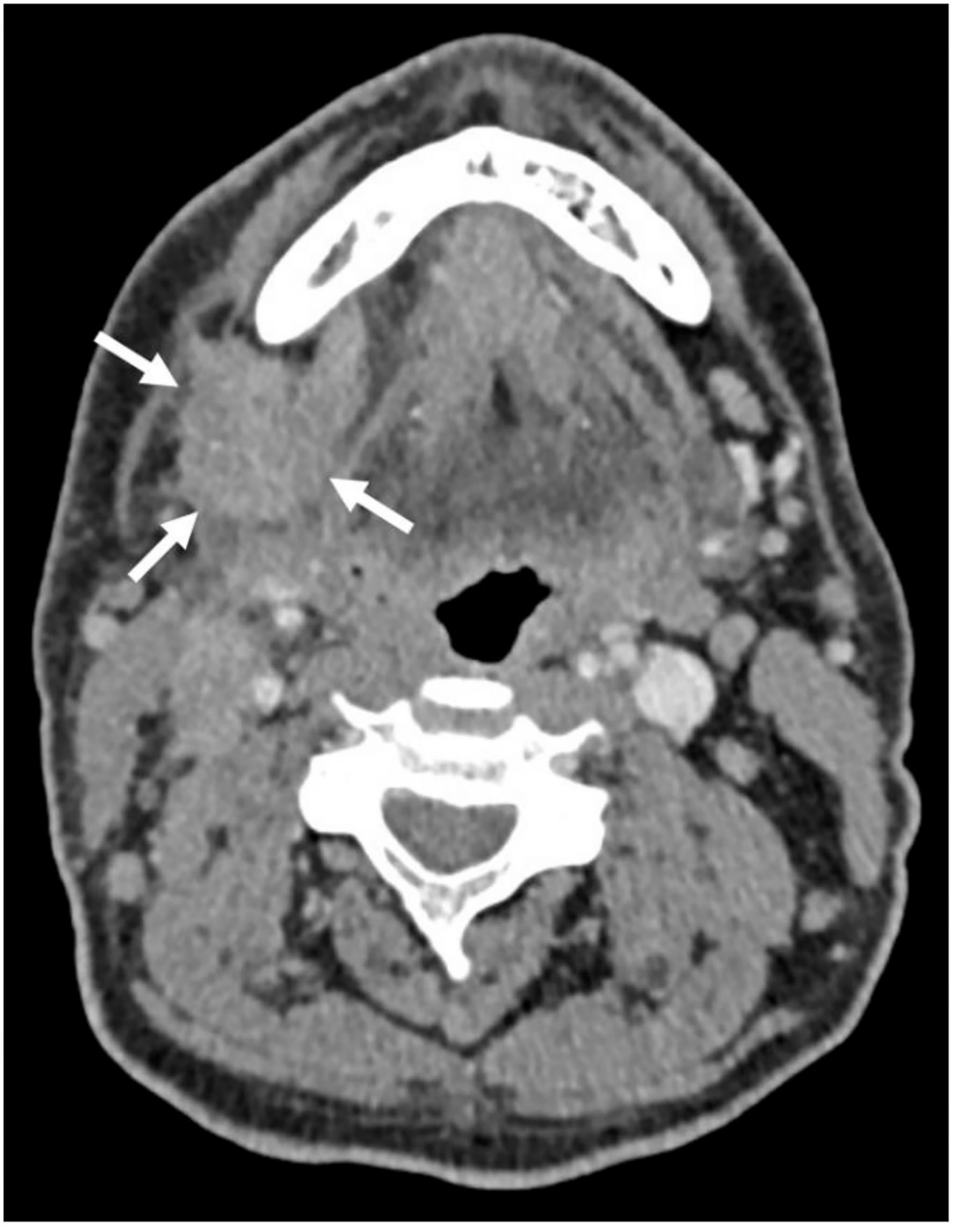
CT image of primary submandibular SDC tumor (indicated by white arrows).
